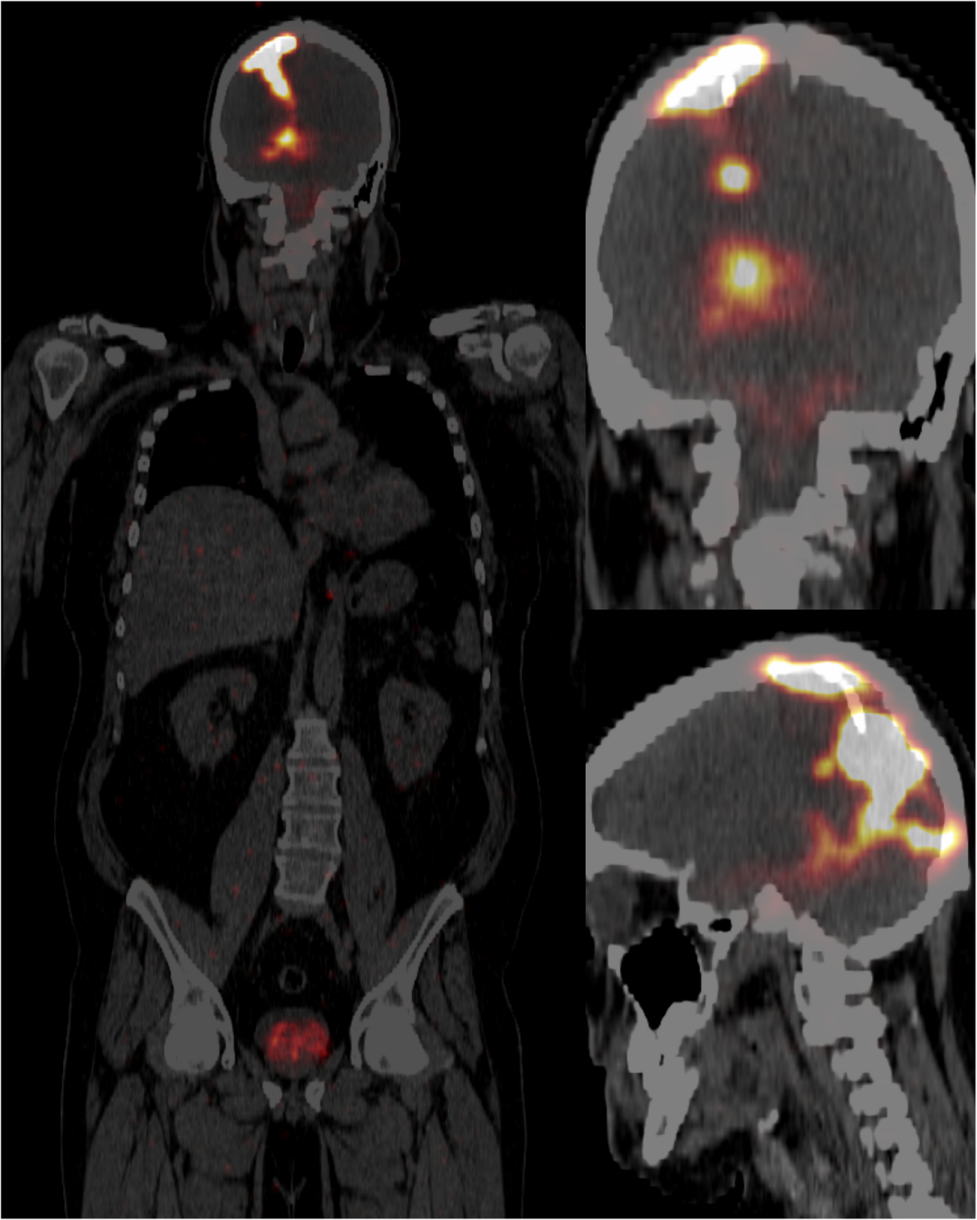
PET/CT after local co-injection of 10-MBq [^68^Ga]Ga-DOTA-SP with a therapeutic dose of [^225^Ac]Ac-DOTA-SP into the resection cavity of a glioblastoma with leakage to the ventriculus. The activity in the bladder was 2.5%
